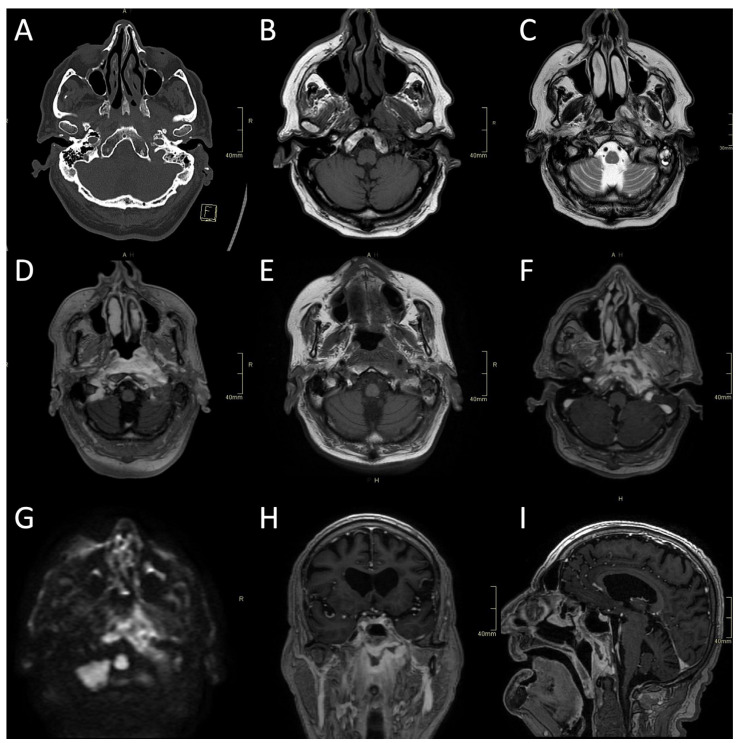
CT and MRI of a fungal atypical skull-base osteomyelitis patient. (A) Axial CT bone algorithm reconstruction showing subtle cortical erosion of the left skull base and the left carotid canal; F—foot. (B) Axial MRI T1 showing subtle asymmetrical hypo-intensity, indicating oedema of the bone marrow on the left side of the clivus. (C) Axial MRI T2 showing asymmetrical hyperintensity in pharyngeal soft tissues and prevertebral muscles, indicating oedema and inflammation. (D) Axial MRI T1 showing the progression of oedema of the bone marrow of the clivus six weeks later. (E) Axial CE MRI showing the progression of hyperintensity in pharyngeal soft tissues and prevertebral muscles and affected bone marrow. (F,H,I) Axial, sagittal, and coronal CE MRI T1 showing extensive inflammatory changes in skull-base bone marrow and soft tissues, with non-enhancing branching fluid collection—abscess in the pre-clival and para/retropharyngeal soft tissues after 11 weeks. (G) Axial MRI DWI showing increased signal in the central skull base. CT: computerised tomography; MRI: magnetic resonance imaging; CE: contrast-enhanced; DWI: diffusion-weighted imaging.
